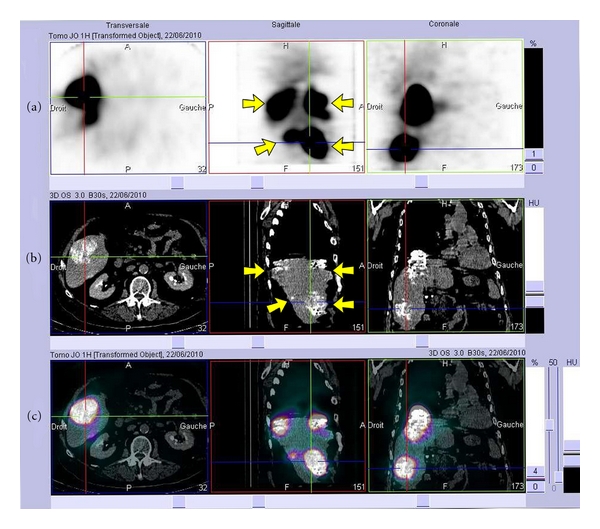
Scintigraphy (a) showing intense hyperfixation (in black) in 4 tumour foci, CT scan (b) showing intense Lipiodol retention (in white) in the nodules and fusion image (c) of scintigraphy and CT scan; 71-year-old male, 1 h after injection of 1.85 GBq of ^188^Re-SSS/Lipiodol. Transverse, sagittal, and coronal views.
